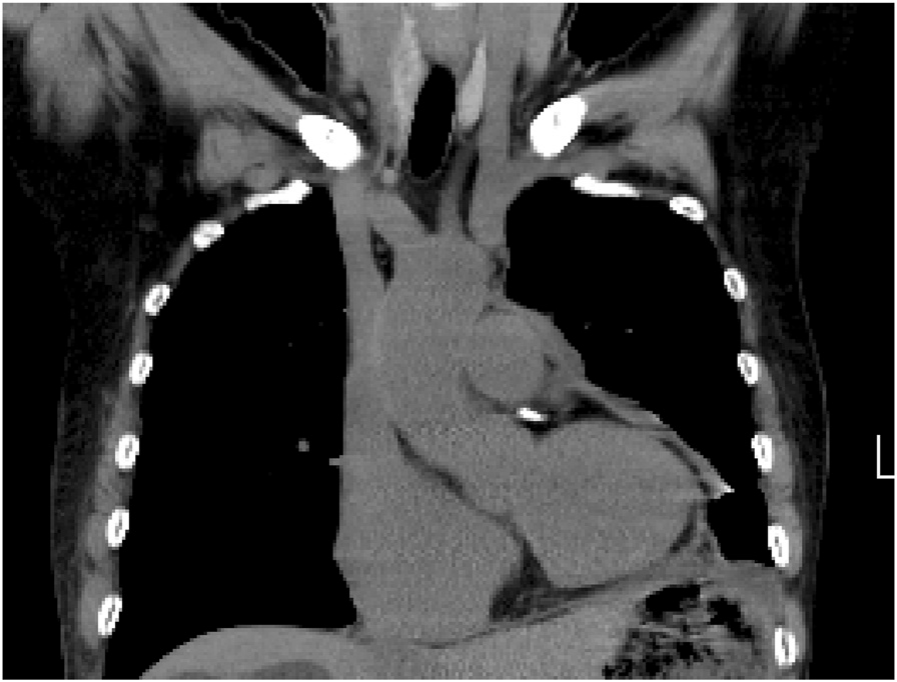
Postoperative computed-tomography images. Computed tomography showed that the pericardial tumor was almost totally resected and there was no evidence of recurrence
